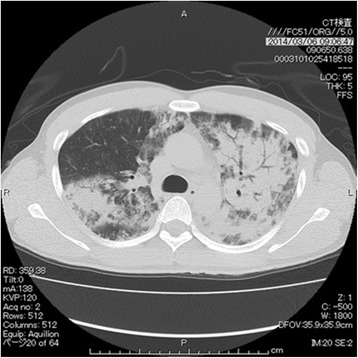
Computed tomography (CT) scan of the lung. CT images showed bilateral infiltration and air bronchograms
